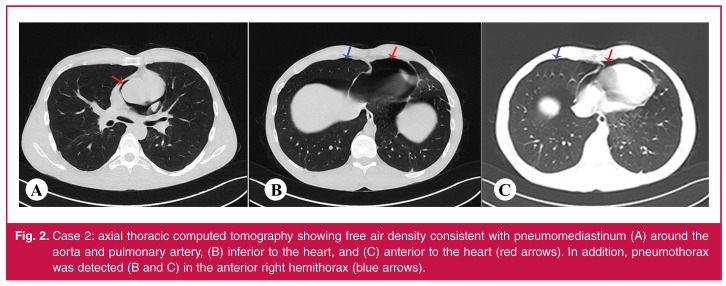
Case 2: axial thoracic computed tomography showing free air density consistent with pneumomediastinum (A) around the aorta and pulmonary artery, (B) inferior to the heart, and (C) anterior to the heart (red arrows). In addition, pneumothorax was detected (B and C) in the anterior right hemithorax (blue arrows).
